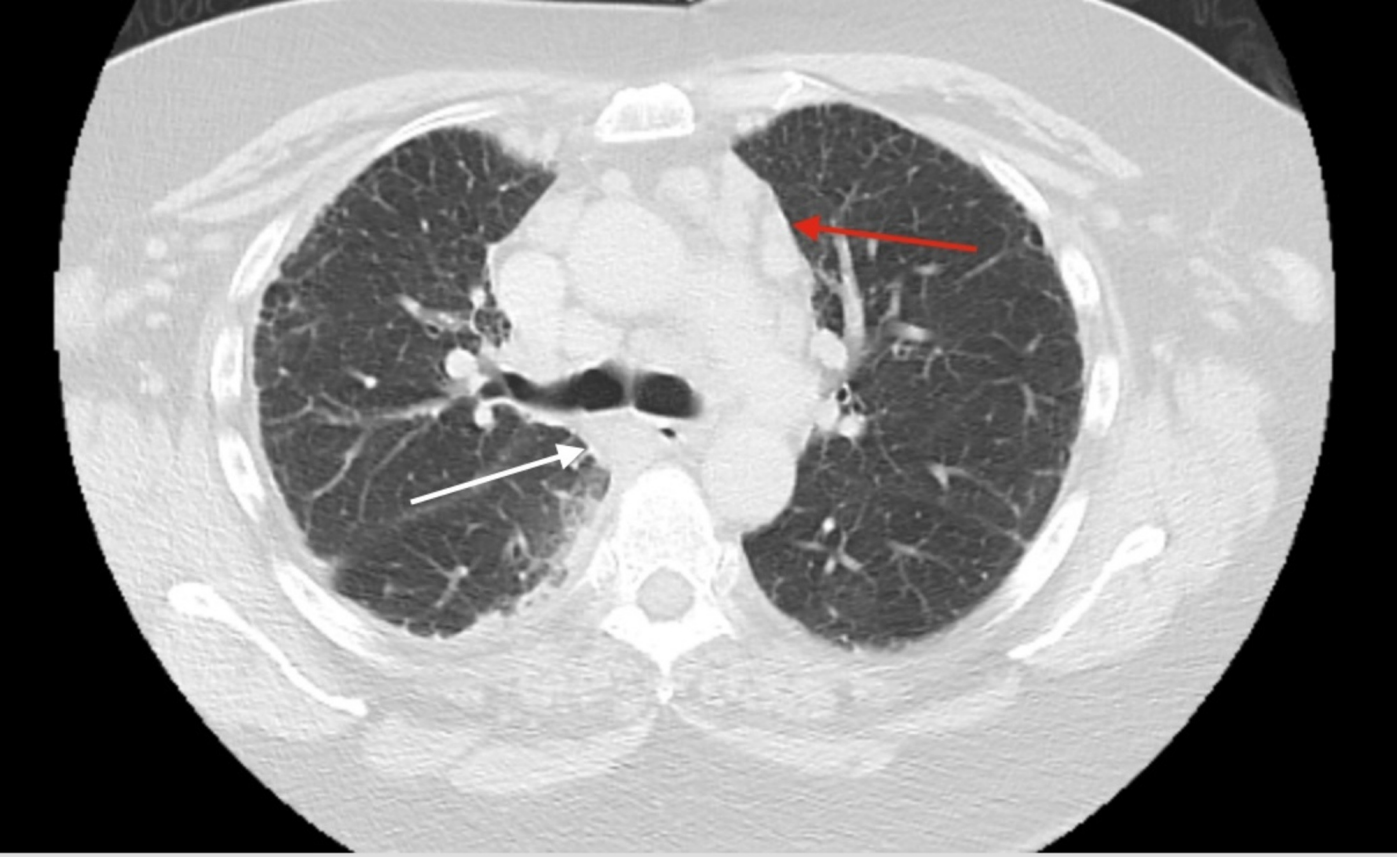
Computed tomography (CT) scan showing enlarged mediastinal and sub-carinal lymph node (see arrows). White arrow: sub-carinal lymphadenopathy Red arrow: mediastinal lymphadenopathy
